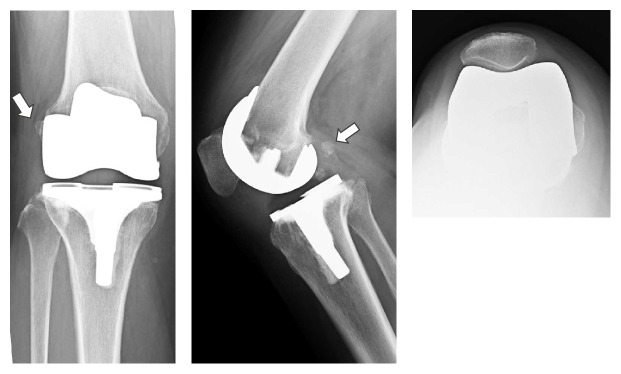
Plain radiographs on first visit; the white arrow identifies the fabella.
